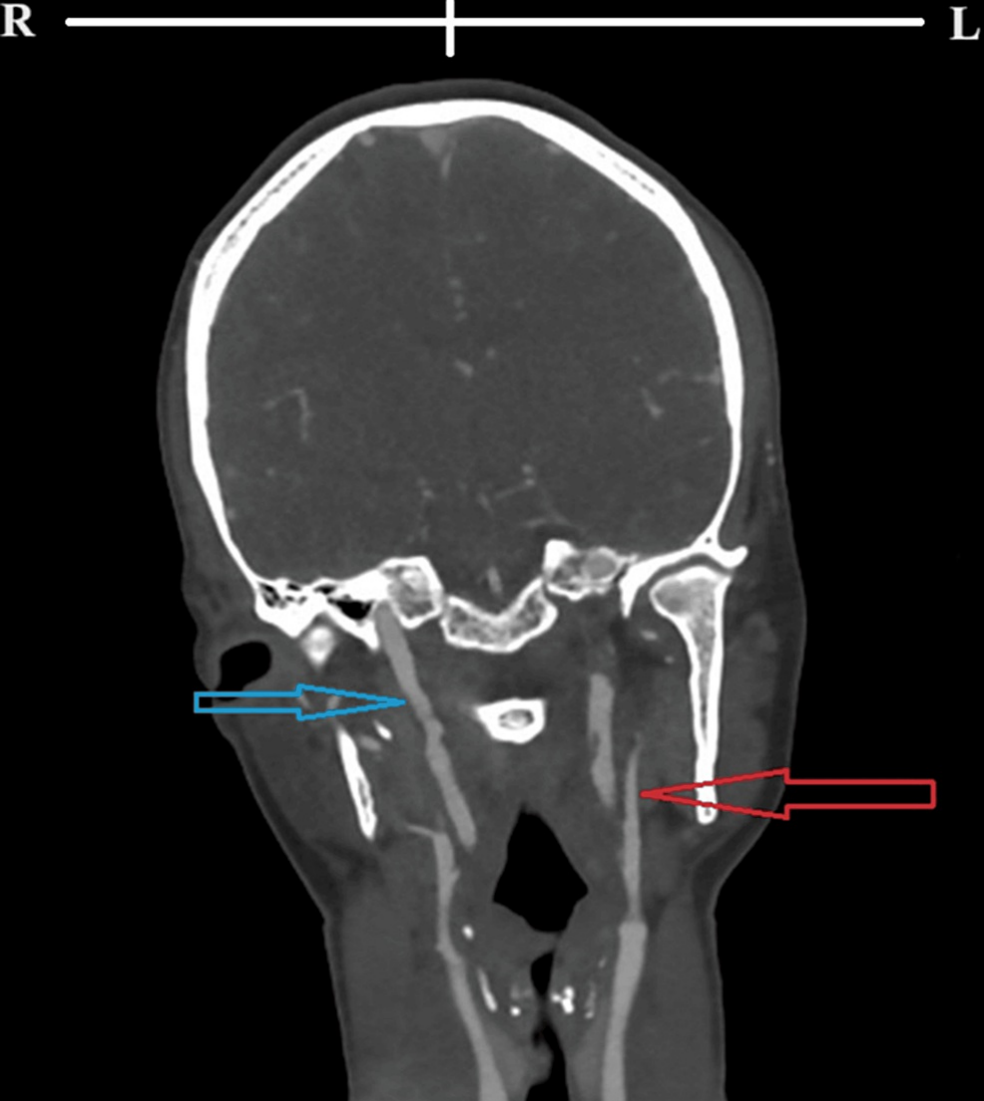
Initial coronal computed tomography angiogram of the neck showing left upper cervical ICA dissection (red arrow) and evidence of arterial intramural thrombus, in addition to stenosis of the upper segment of right ICA (blue arrow) R: right side; L: left side; ICA: internal carotid artery
